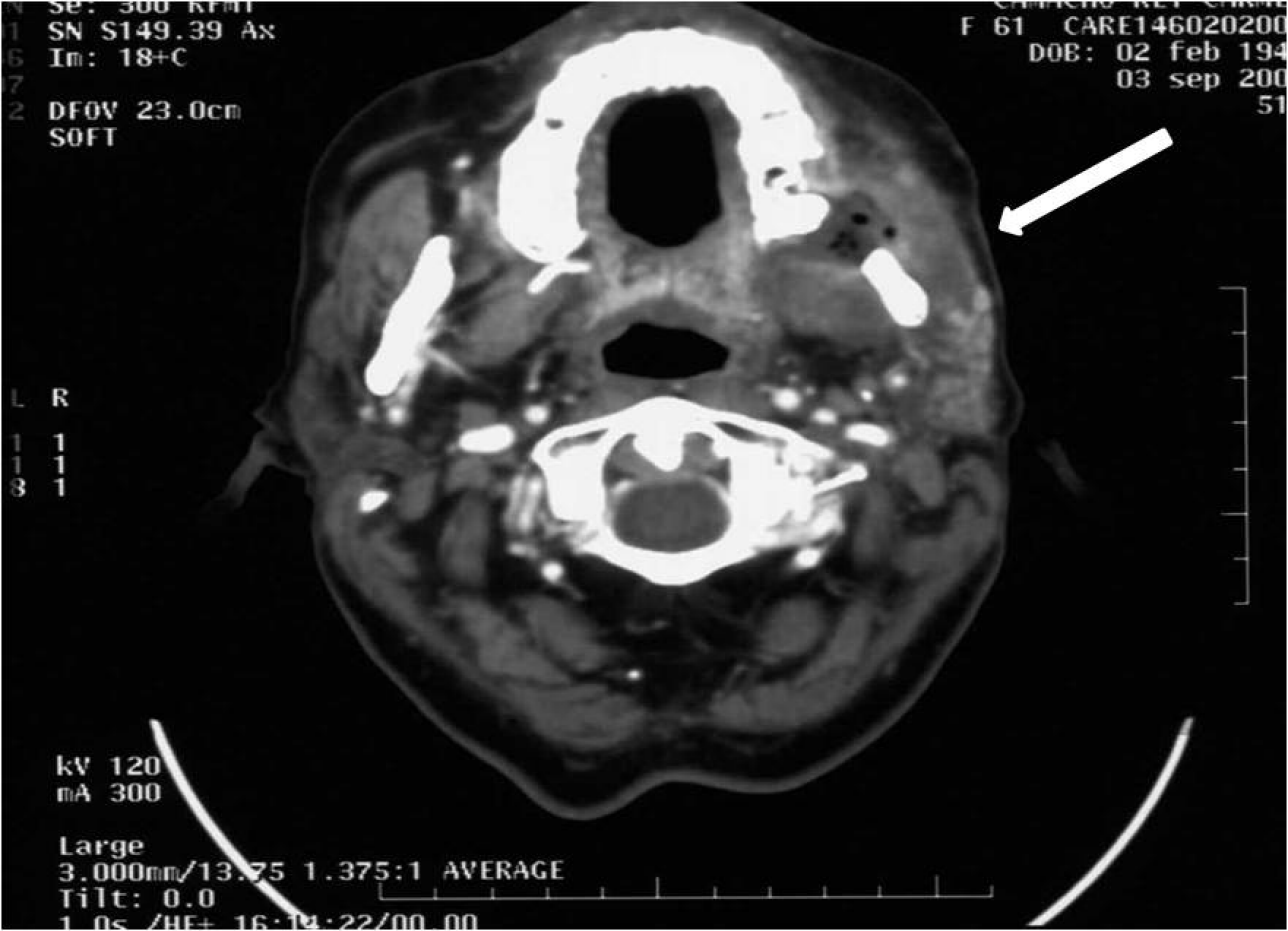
Axial contrast-enhanced cervical computed tomography scan after 3 cycles of cetuximab plus platinum-based chemotherapy. The scan shows a marked reduction in the tumor. A large hypodense area with air bubbles and peripheral enhancing corresponding to necrosis is seen in the masticator space. A partial tumor remission was considered.
